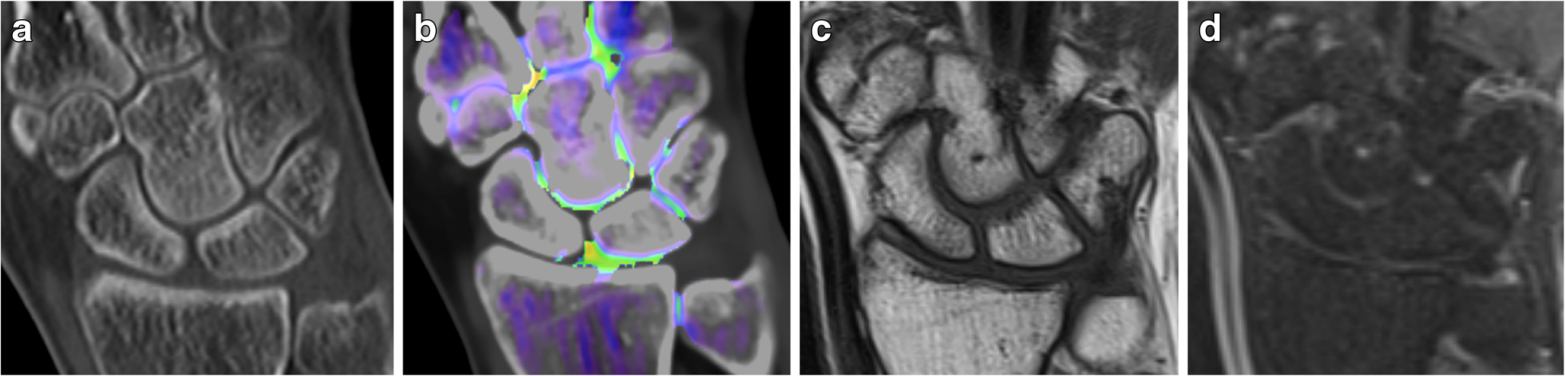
A 40-year-old female with a clinical suspicion of scaphoid fracture, but no fracture line seen on native CT (Fig. 3a) or any sign of BME on VNCa imaging (Fig. 3b) or MRI with coronal T1-weighted (Fig. 3c) and fluid-sensitive (Fig. 3d) sequences
